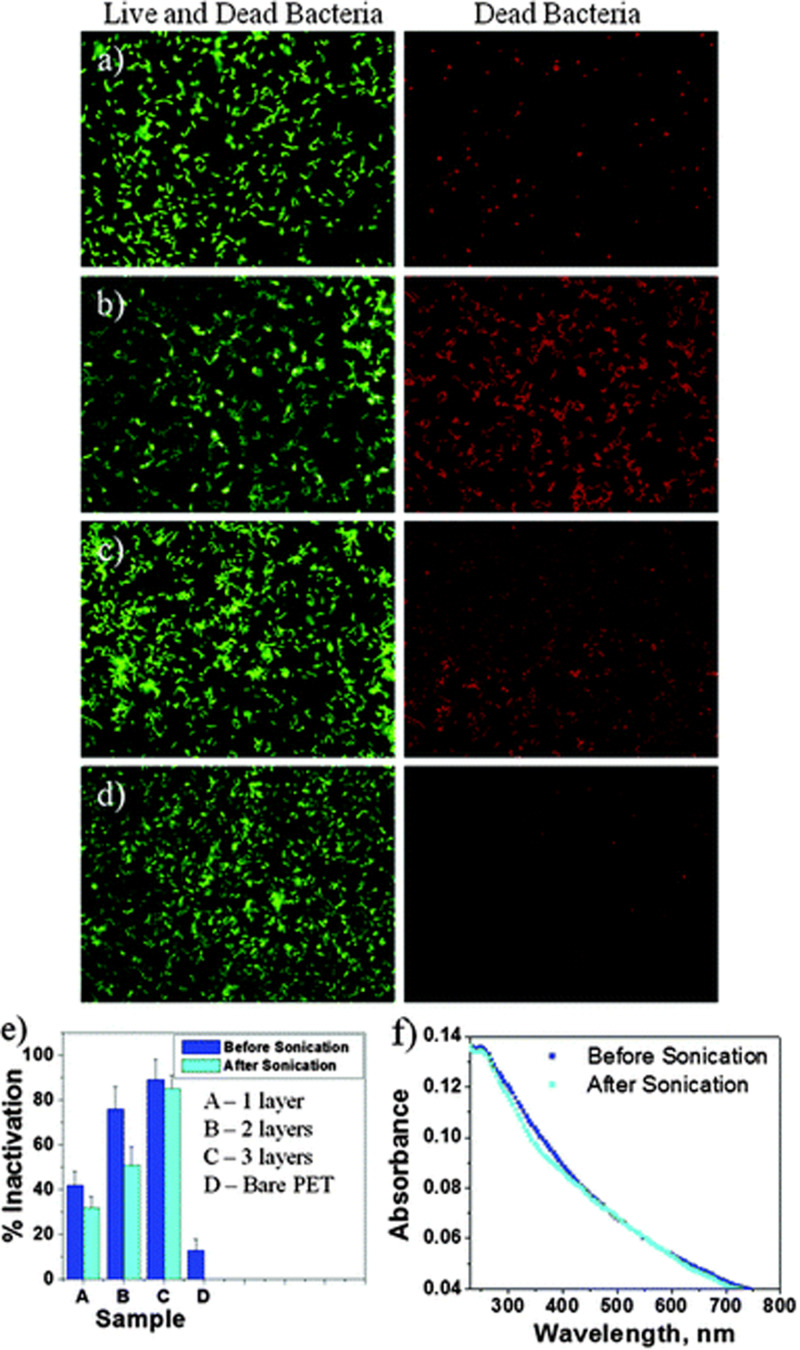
Representative fluorescence images of *E. coli* on a single (a), double (b), and triple (c) layers of GO-LB and bare PET (d). (e) Comparison of the antibacterial effect before and after ultrasonication. (f) UV-Vis absorbance of 3-layer GO-LB film before and after ultrasonication. Reproduced with permission from Akhavan et al. (2012).
